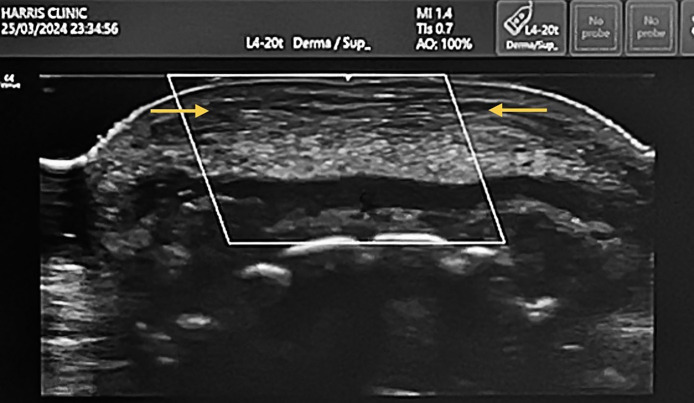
High-frequency ultrasound of the lower lip showing unintended intramuscular (pars marginalis) hyaluronic acid filler deposition (between arrows), consistent with chronic overfilling patterns.
